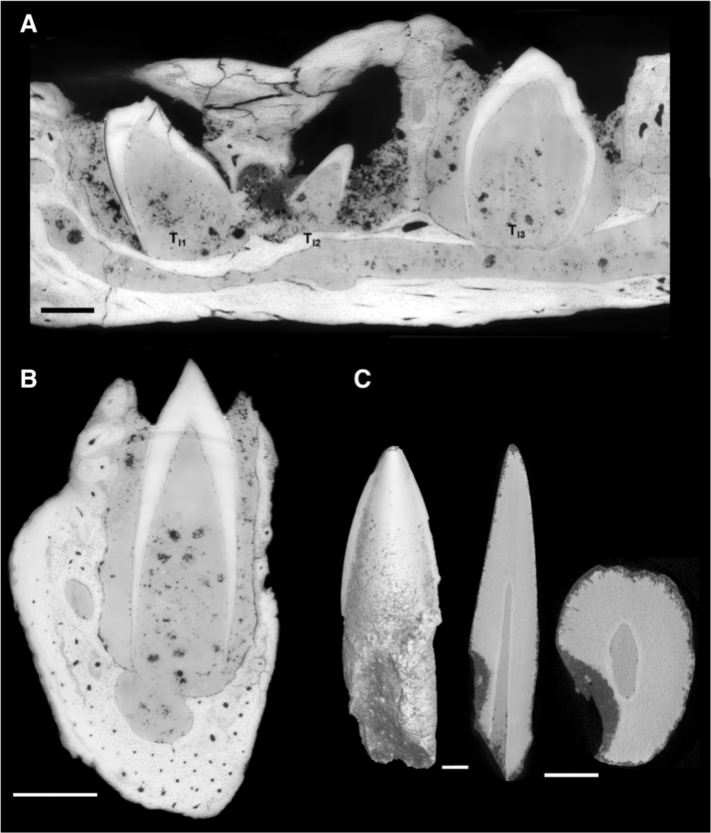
X-ray microtomographic images showing *Ichthyornis* tooth implantation and replacement. a Parasagittal view of the *Ichthyornis dispar* dentary fragment YPM.1775 with teeth inserted in sockets. The alveolar space is filled with sediment and there is no attachment tissue or preserved cementum around the root of the teeth. b Transverse section of the *Ichthyornis dispar* dentary with T_I3_ tooth. c
